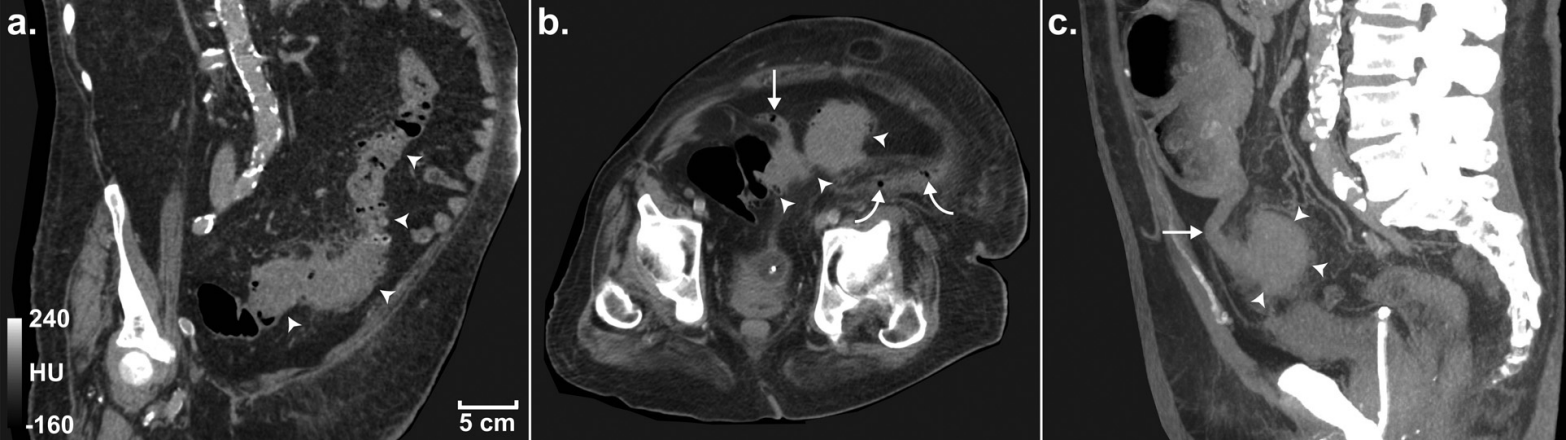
Admission CT images showing sigmoid diverticulitis, appendicitis and locules of free gas in the left retroperitoneum. (A) 1.25 mm thick coronal oblique slice demonstrating a long segment of thickened sigmoid and descending colon (arrowheads) with multiple inflamed diverticula; (B) 1.25 mm thick axial slice demonstrating the inflamed appendix (arrow) adherent to the inflamed sigmoid (arrowheads) and locules of gas within the retroperitoneal space (curved arrows) and (C) a sagittal, 30 mm thick maximum intensity projection demonstrating the position of the appendix (arrow) adherent to the sigmoid colon. All images have a window width and level of 400 and 40, respectively, and the in-plane resolution of all images is 0.63 mm.
